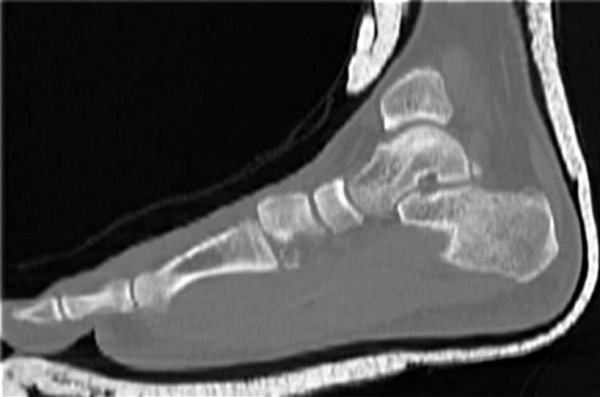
Computed tomography of the left foot. There is disruption of the tarsometatarsal (Lisfranc) joint with associated soft tissue swelling.
